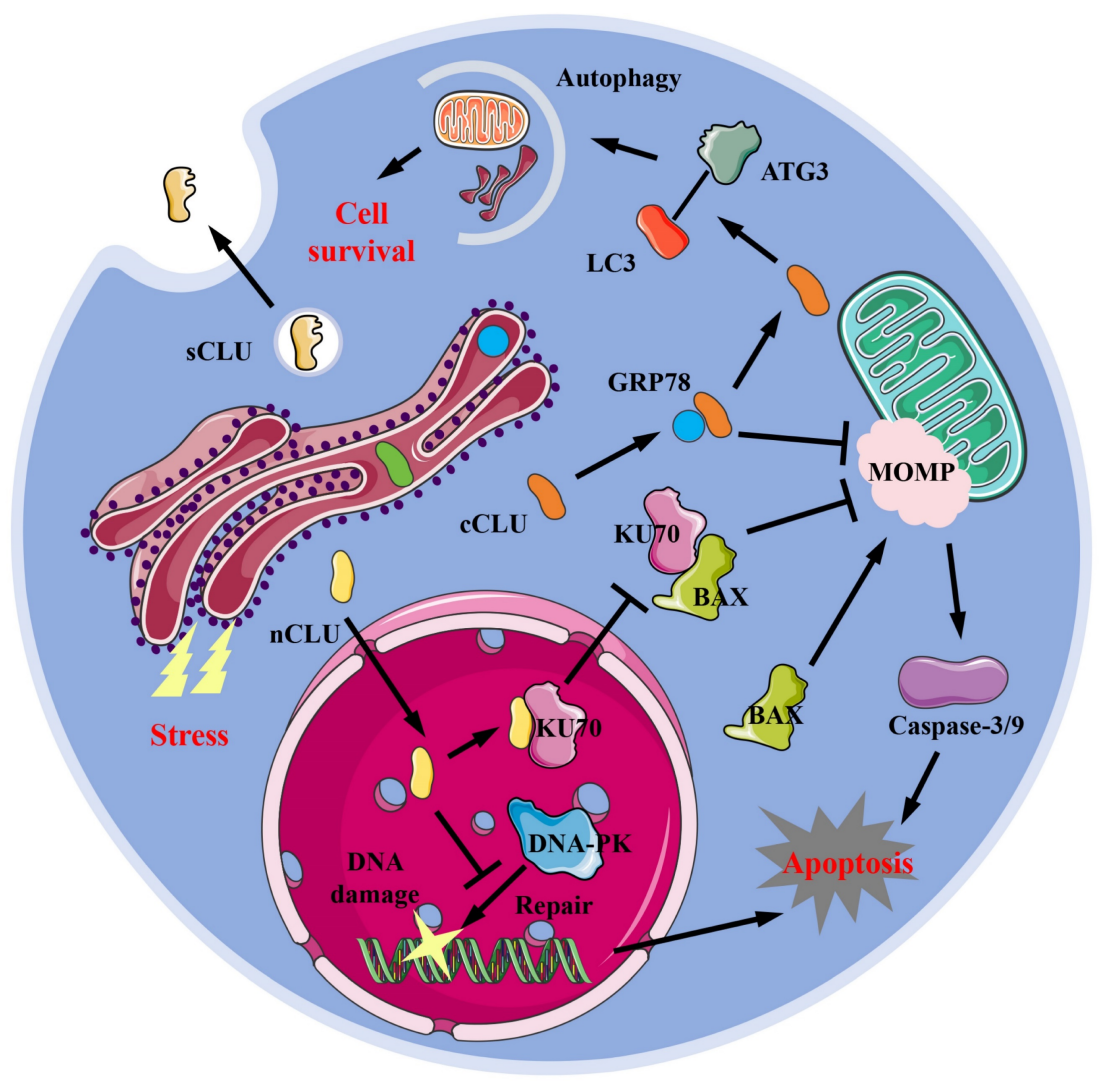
Forms and potential mechanisms in physiological functions of CLU protein. sCLU, secreted type clusterin; cCLU, cytoplasmic type clusterin; nCLU, nucleus type clusterin; LC3, microtubule-associated protein 1 light chain 3; ATG3, autophagy-related protein 3; GRP78, glucose regulated protein 78; MOMP, mitochondrial outer membrane perforation; KU70, X-ray repair cross complementing 6; BAX, BCL2 associated X; DNA-PK, DNA-dependent protein kinase.
